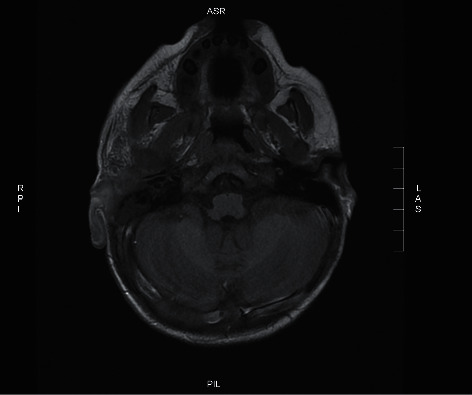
T2 MRI with no contrast showing significant inflammatory changes in the right ear canal, periauricular region.
